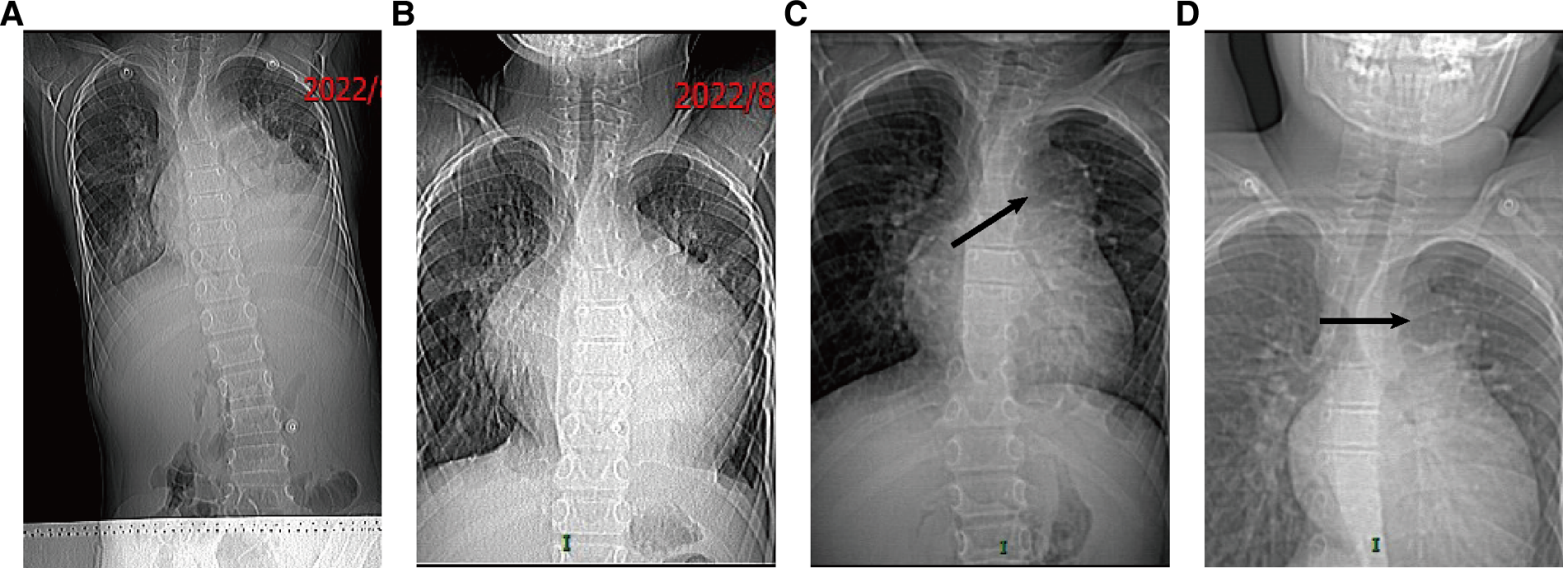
The follow-up chest x-ray from August to November: (A) chest x-ray on 10 August 2022; (B) chest x-ray on 21 August 2022; (C) chest x-ray on 16 September 2022; (D) chest x-ray on 14 November 2022.
